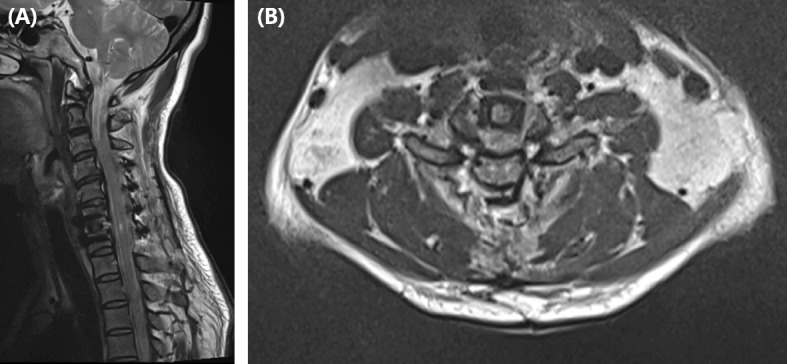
a One week postoperative sagittal T2 weighted MR image showing high intensity patchy signal changes within the cord with cord swelling as called White cord syndrome. b One week postoperative axial T2 weighted MR Image showing 2 points high signal intensity at C6–7 level
